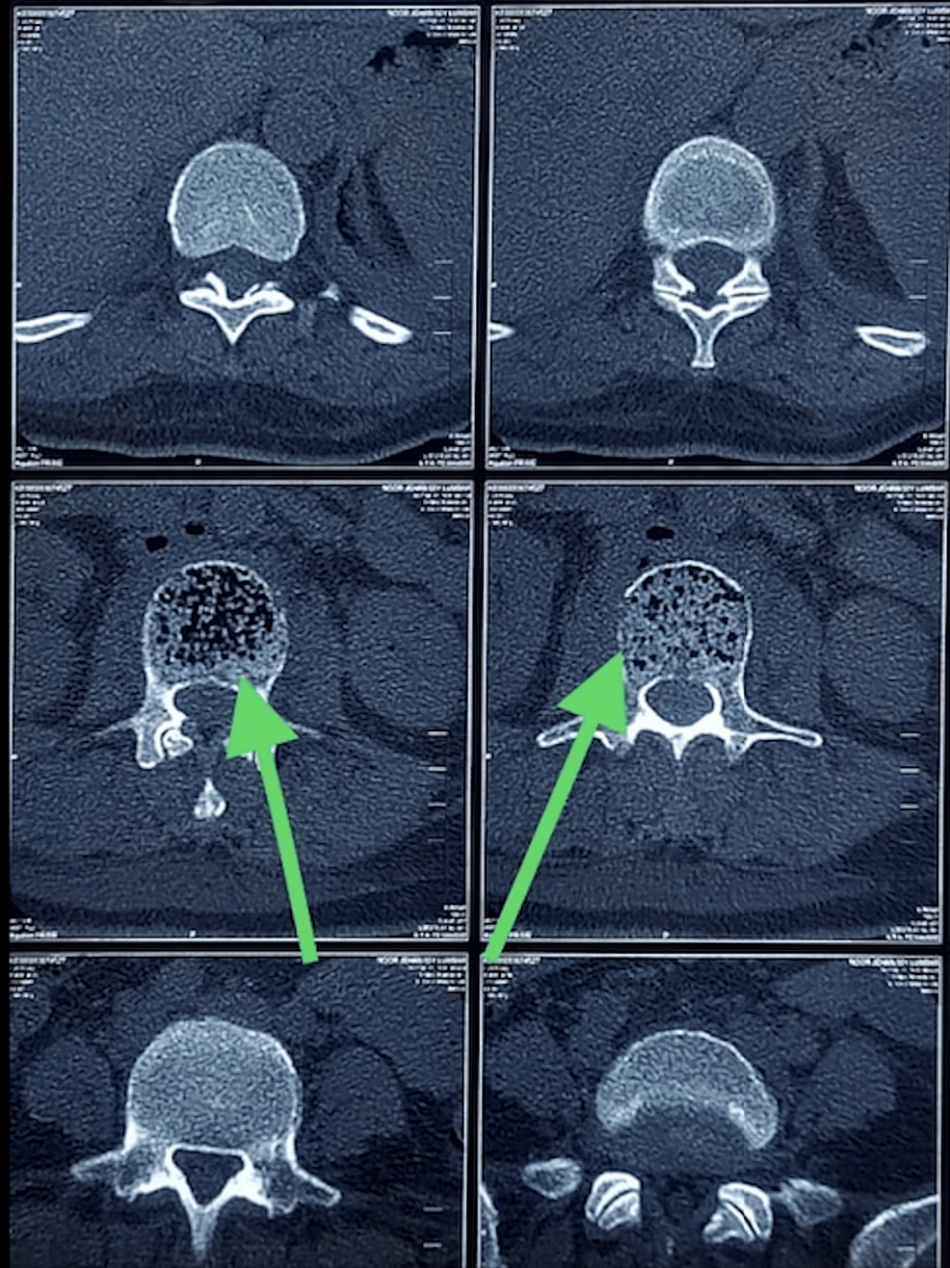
CT scan of the spine in the coronal plane view shows intraosseous air foci at the L3, indicated by the arrow. CT: computed tomography, L3: third lumbar spine vertebra
